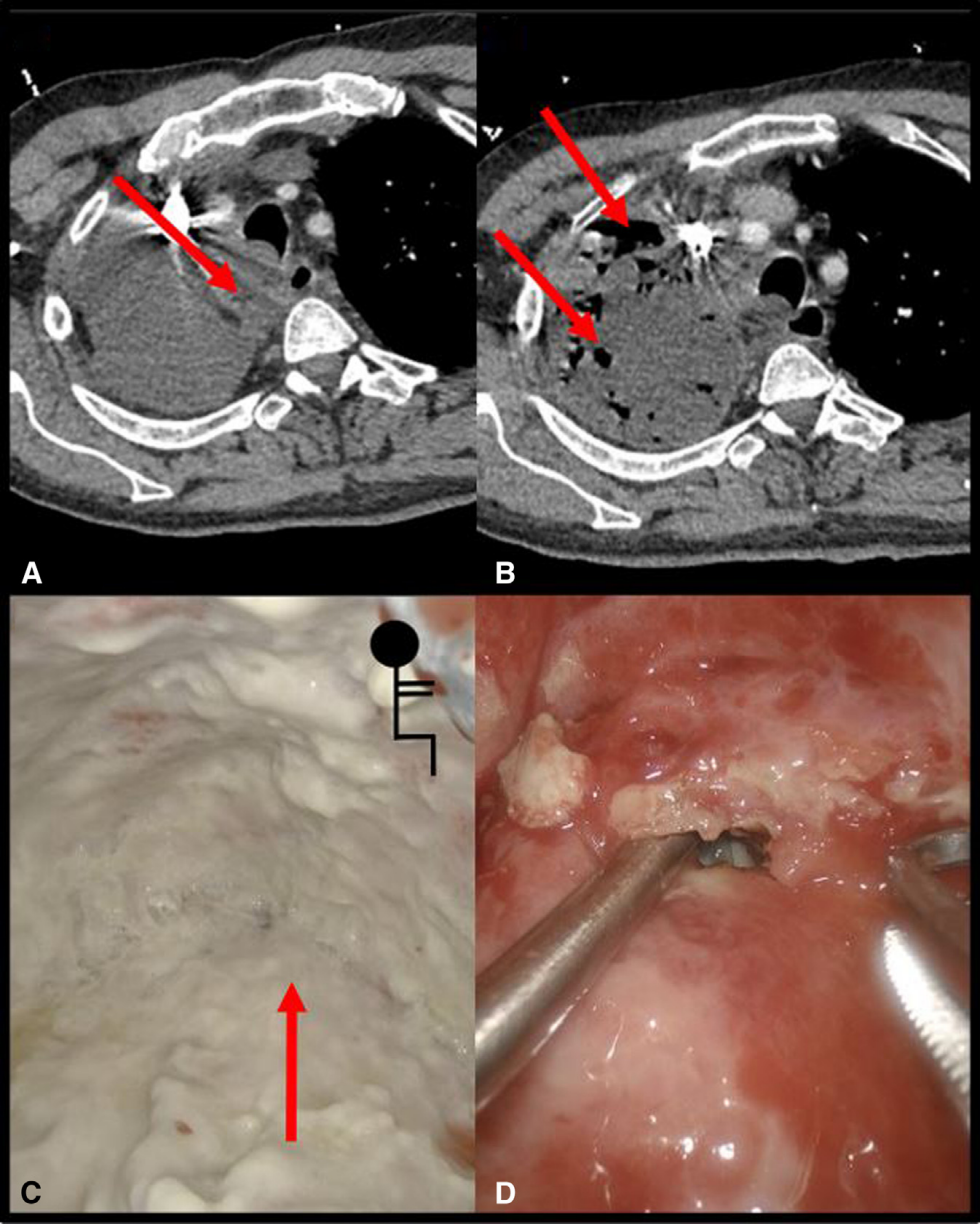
Radiographic and intraoperative clinical findings. A, Contrast-enhanced axial CT 10 days after the procedure identifying right paratracheal fluid collection. B, Contrast-enhanced axial CT 17 days after the procedure identifying progression of right paratracheal fluid collection and postpneumonectomy space gas. C, Intraoperative photograph of the right thoracic cavity with diffuse fibropurulent material throughout the chest. *Arrow* indicates the paratracheal mediastinal abscess before irrigation. D, Intraoperative photograph of the right paratracheal mediastinal abscess cavity in communication with the right pleural cavity. *CT*, Computed tomography.
